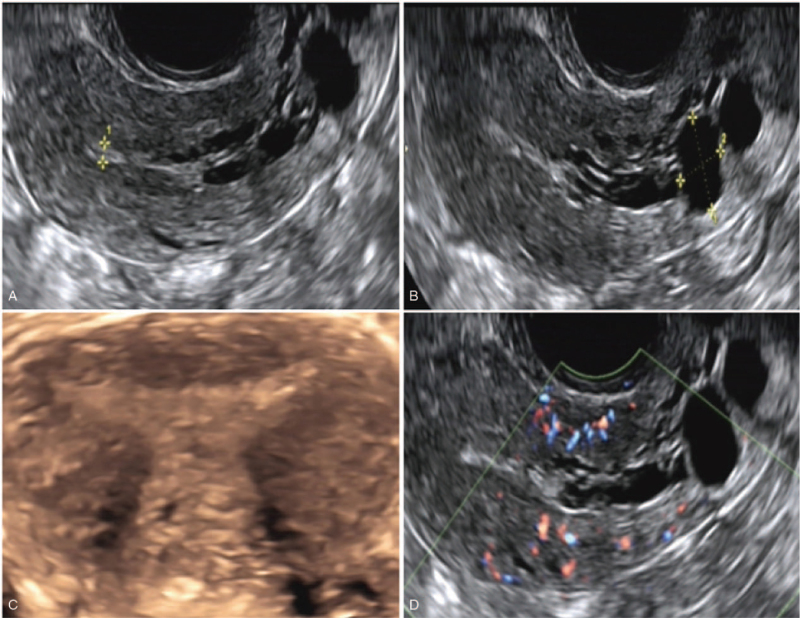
Vaginal ultrasound evaluation on the 16^th^ day of menstruation: ROF: 19.2 mm, LOF: 3 mm, and average intima thickness: 8.8 mm; endometrial 3D color Doppler: multiple cystic echoes in the middle and lower endometrium. LOF = left ovary follicle, ROF = right ovary follicle.
